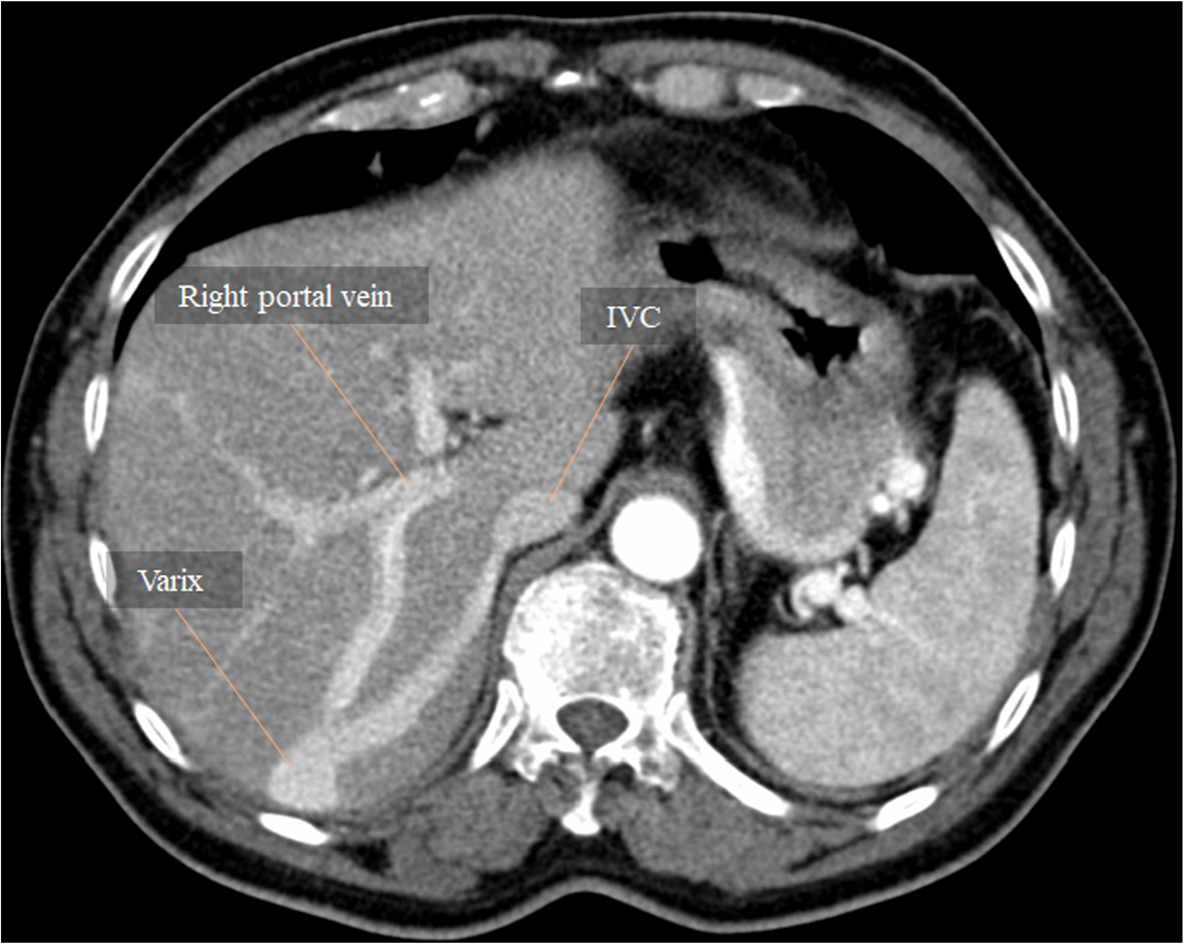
Congenital intrahepatic portosystemic shunt. MPR image from late arterial phase CT displaying the most common type of congenital intrahepatic portosystemic shunt (type 1), with a single vessel connecting the right PV with the IVC. Note the large caliber of both afferent PV branch and efferent hepatic vein, and the presence of a variceal dilatation between both, appearing as a rounded enhancing mass. If considering this focal varix as an aneurysm, we can classify this shunt as a type 3 either
